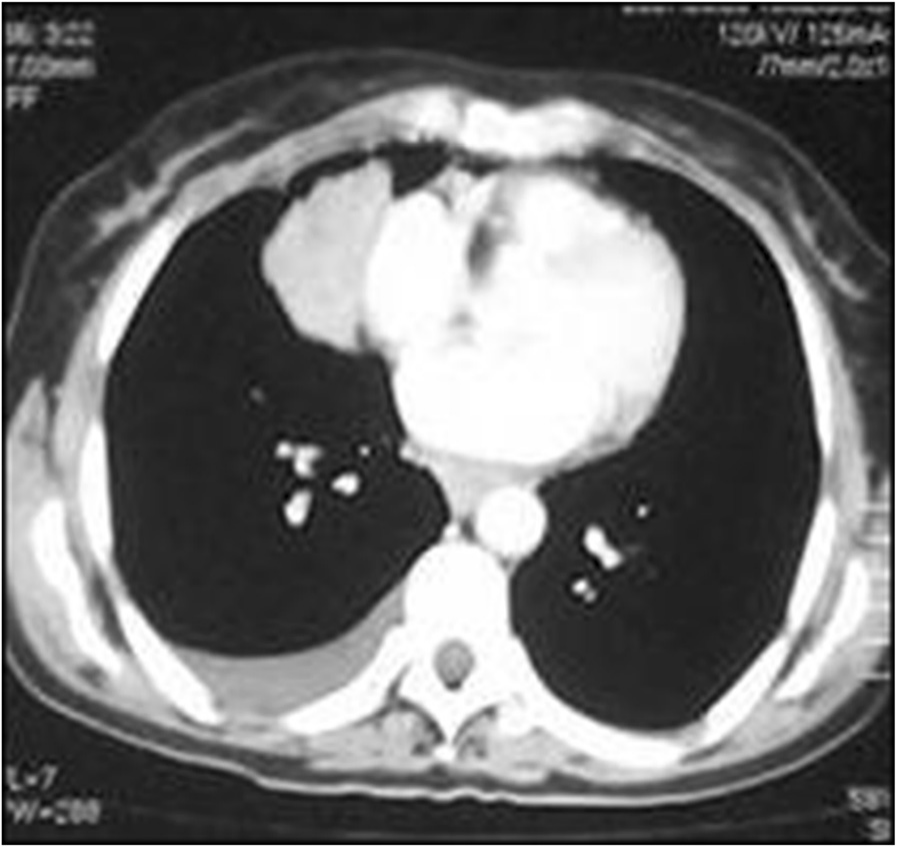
CT showing a right paracardiac intrapulmonary mass lesion and pleural effusion.
